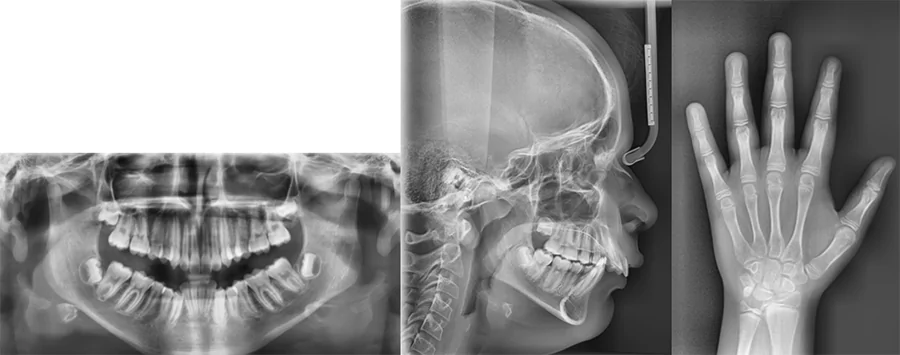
Pretreatment panoramic, cephalometric, and hand-wrist radiographs
